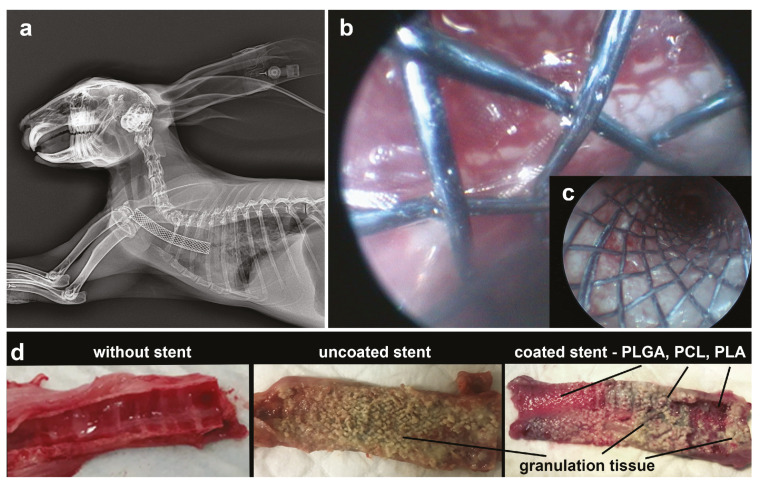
X-ray (a) and endoscopic images (b,c) during stent implantation surgery. On endoscopic images, the film with microchamber arrays is visible (b). Photo of the normal trachea and trachea 10 days after stent implantation with and without coating (d).
